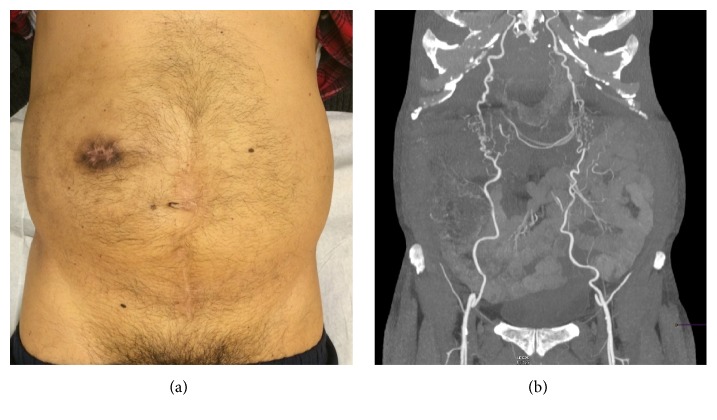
Postoperative view of the patient's abdomen, compared to a preoperative CT angiography, showing the proximity of Winslow's pathway and of the ileostomy.
